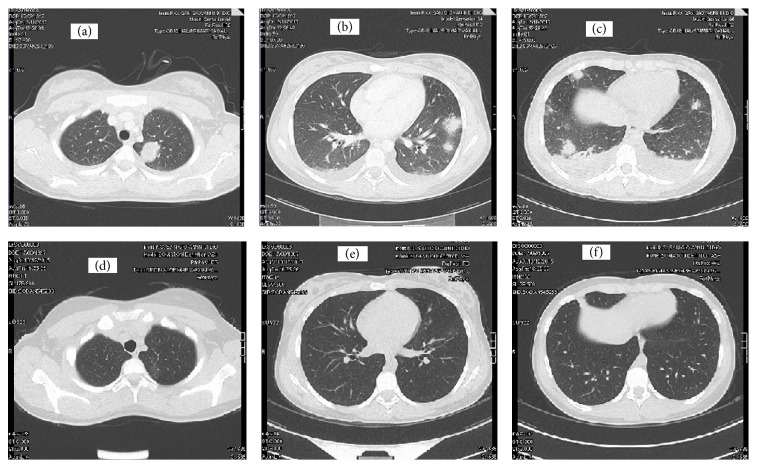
(a, b, c) CT scan of the thorax demonstrating bilateral nodular lesions and bilateral pleural effusion. (d, e, f) CT scan after 4-week antibiotic therapy which shows the full resolution of the nodular lesions and pleural effusion.
